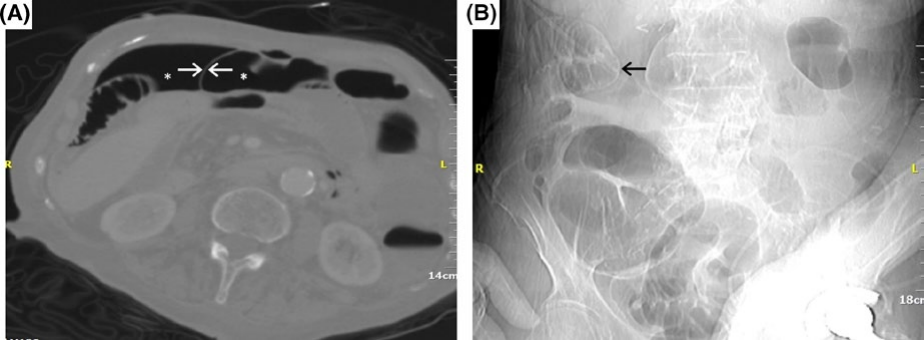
A, Abdomen computed tomography (IV contrast lung window). Free abdominal gas (White stars) and falciform ligament (White arrows). B, Plain abdominal radiograph. Clear delineation of small bowel wall at right upper quadrant, due to free intraabdominal gas (Black arrow)
